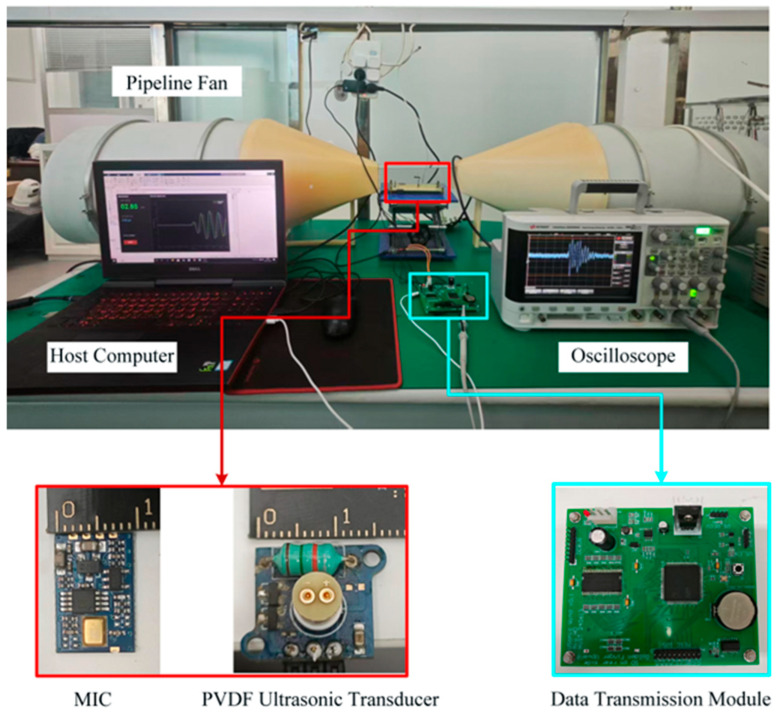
PVDF-based ultrasonic wind speed measurement system and wind-tunnel testing environment.
